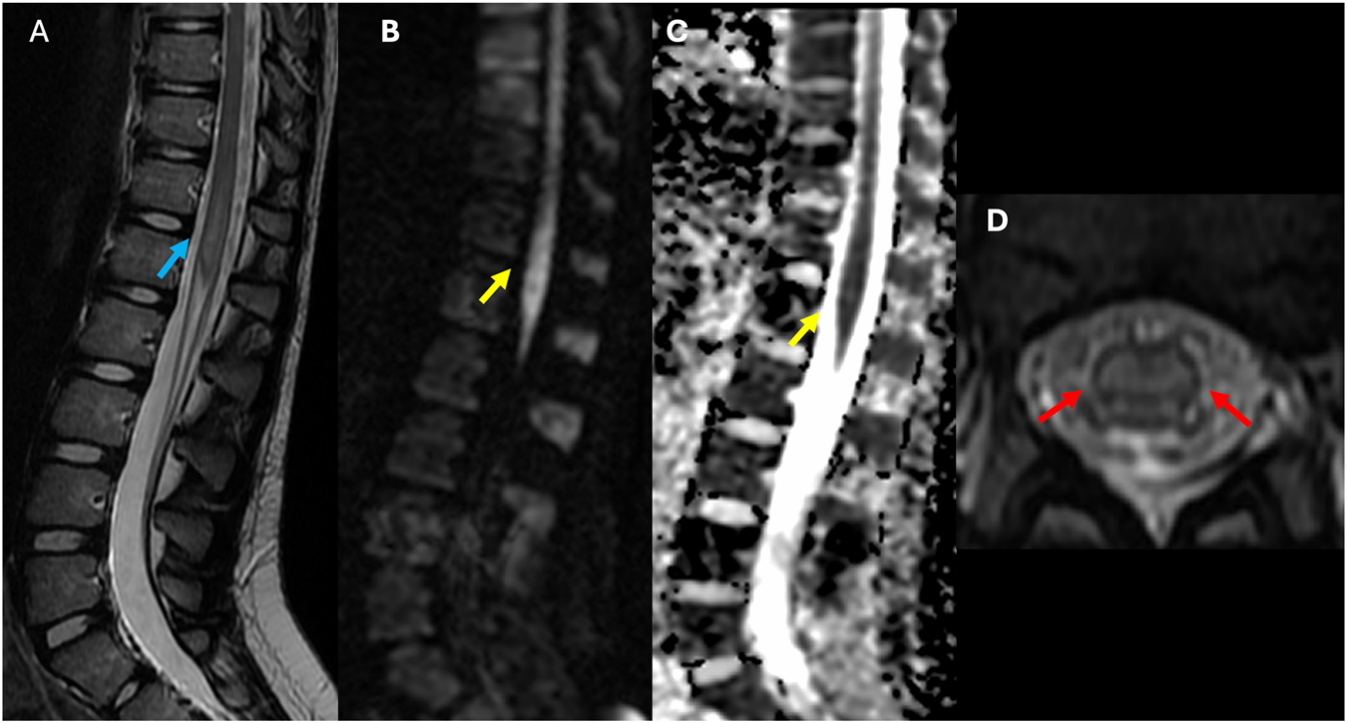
Forty-one-year-old male who presented with acute lower extremity weakness and back pain. A Sagittal T2 image of the lumbar spine demonstrates mild expansion and diffuse abnormal high signal intensity within the distal cord and conus medullaris. (blue arrow). B (DWI) and C (ADC) showing diffusion restriction in the conus medullaris (yellow arrow). D Axial T2 image of the lumbar spine demonstrates expansion and centrally located high signal intensity within the conus medullaris (red arrow)
